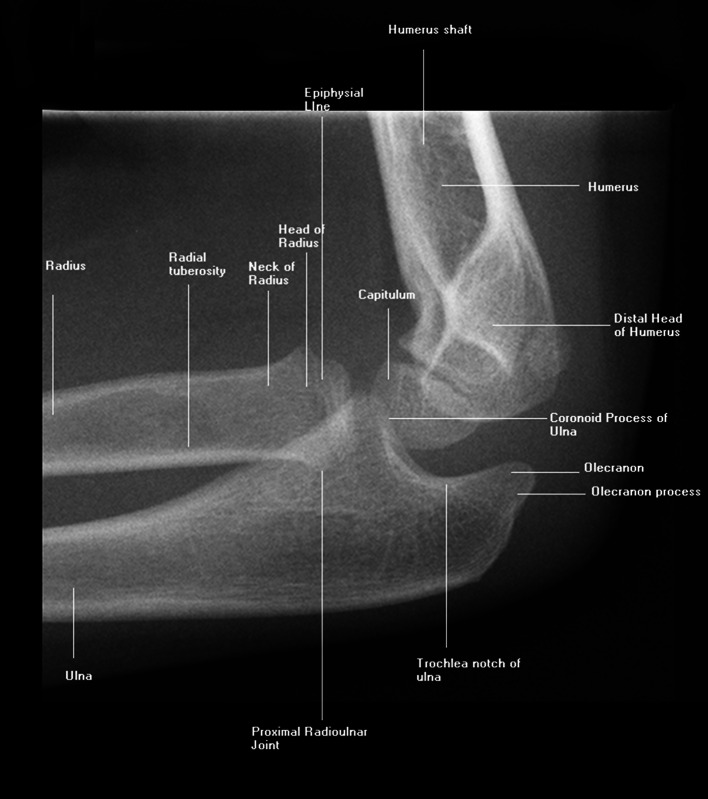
Typical pediatric elbow radiograph. The radiologic anatomy of a pediatric elbow showing some of the features that would be considered in searching for an abnormality such as a fracture
